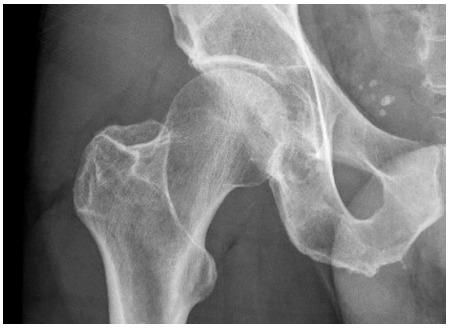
Case 1: X-ray of the right hip on the day of injury
